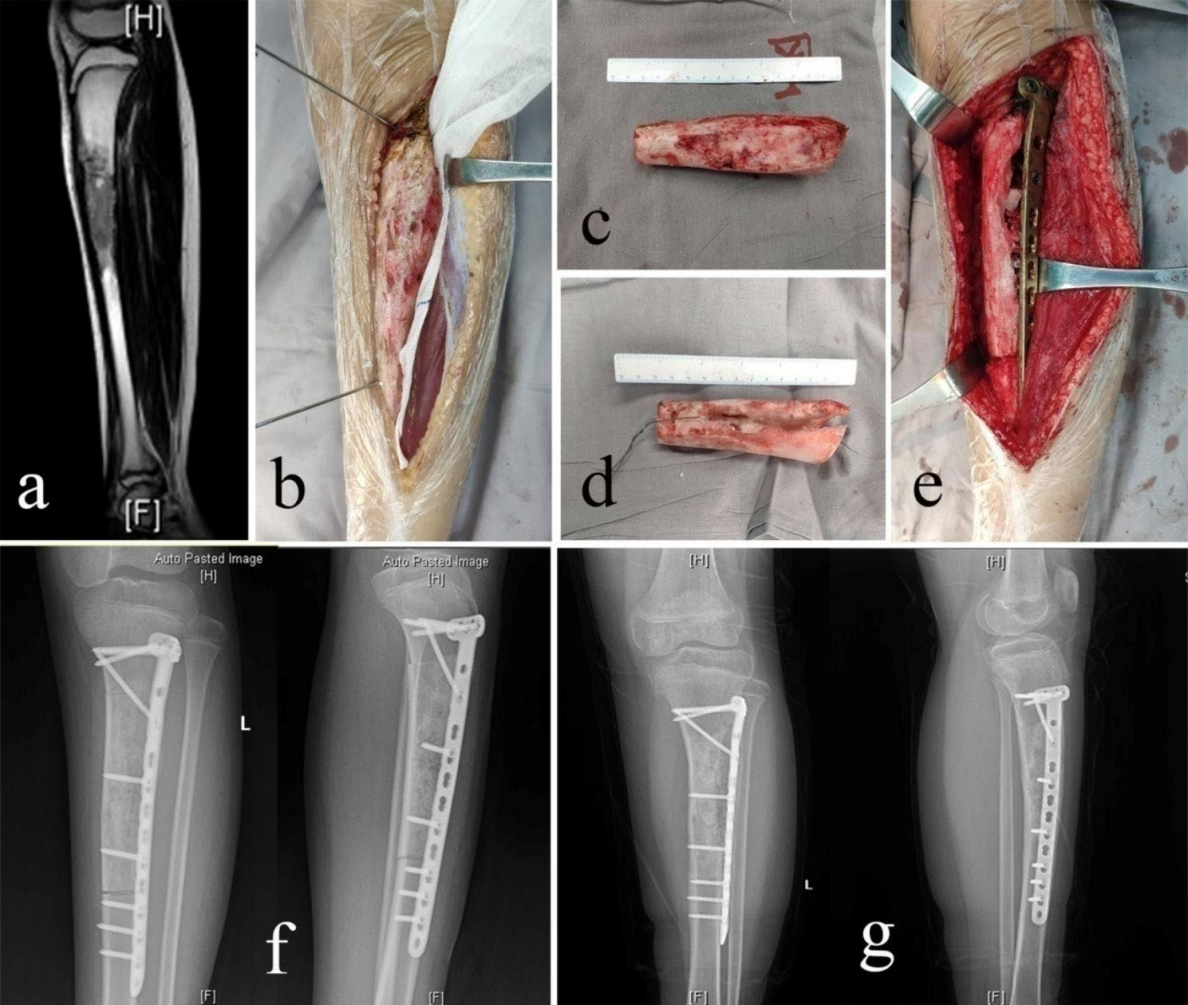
A 14-year-old female with osteosarcoma involving the diaphysis of the left tibia (case 19). (a Preoperative T2-weighted MRI (TR 2508.5ms, TE 100.0ms) showed a tumor located in the diaphysis of the left tibia. b Intercalary resection using a wire saw. c Resected bone. d Resected bone treated with liquid nitrogen. e The frozen autograft was reimplanted and fixed with a locking plate and screws. f Postoperative X-rays. g X-rays at 5 months after reimplantation showing union at both osteotomy sites.)
